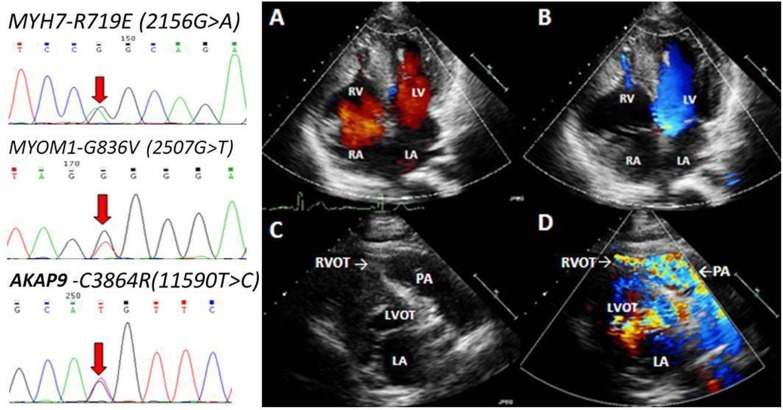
Transthoracic echocardiography and gene sequencing. An apical 4-chamber view of an 18-year-old patient with HCM demonstrating pronounced hypertrophy of the RV free wall during end-diastole (A) and RV cavity obliteration in the mid-ventricular region, as well as in the apical cavity (B). (C) Modified parasternal short-axis views with color Doppler flow mapping demonstrating striking hypertrophy of the RV anterior wall and narrowing of the RV outflow tract. (D) Color Doppler mapping demonstrating turbulent flow from the RV outflow tract to the PA (arrow). In the left panels, the red arrows indicate the double-peak at the site of the identified mutations. LA: left atrium; LV: left ventricle; LVOT: left ventricular outflow tract; PA: pulmonary artery; RA: right atrium; RV: right ventricle.
